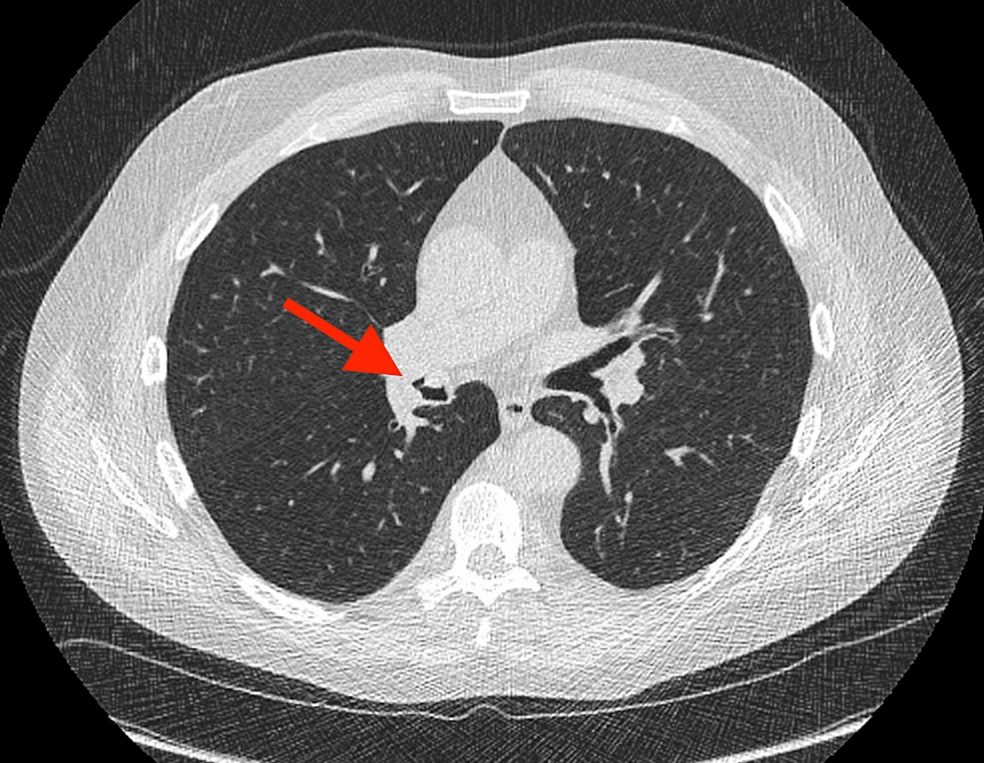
An axial image of the low-dose chest CT without intravenous contrast performed two years prior, with the endobronchial lesion again seen within the bronchus intermedius (red arrow), apparently smaller in size in comparison to the more recent study.
